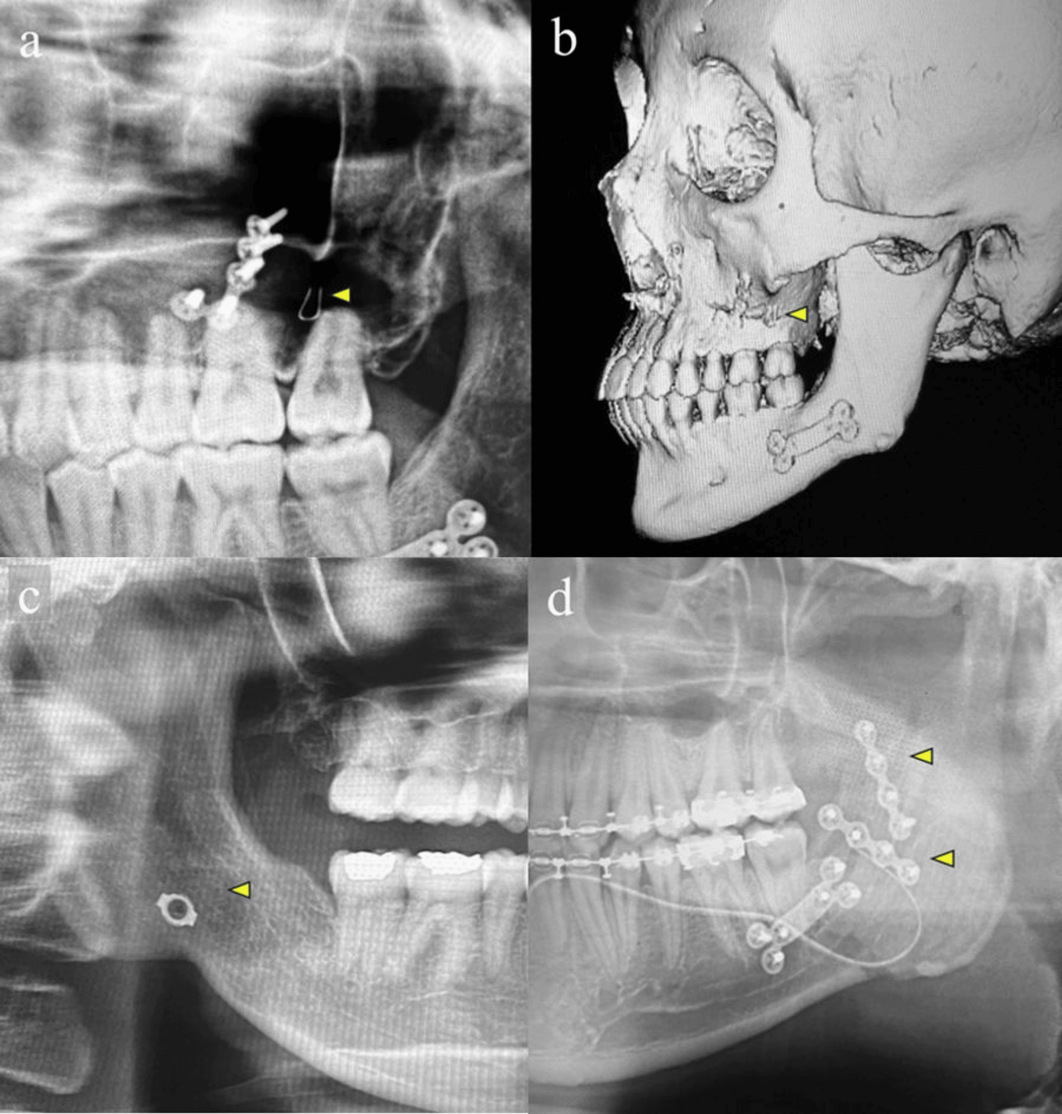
Representative cases of complications following orthognathic surgery SW monofilament testing instruments for sensory evaluation Figures 2a, 2c, 2d are panoramic X-ray images, and Figure 2b is a 3D multi-detector computed tomography (MDCT) reconstruction. Case 1 (a, b): Inadvertent retention of a metal wire for intermaxillary fixation along the buccal bone wall of the left posterior maxilla. (a) A panoramic radiograph showing the retained metal wire (arrowhead). (b) Three-dimensional MDCT reconstruction showing the same retained metal wire (arrowhead). Case 2 (c): Inadvertent intrusion of the cut edge of a metal fixation plate into the lateral space of the mandibular ramus (arrowhead). Case 3 (d): Unfavorable split(s) of the anterior margin of the mandibular ramus (arrowheads).
